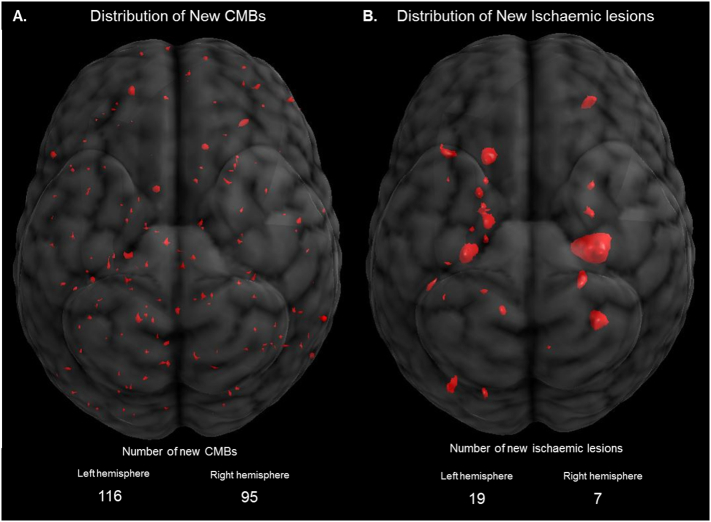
Positions of (A) new cerebral microbleeds, and (B) new MRI FLAIR lesions seen in the combined imaging data for all 46 patients. Only 4 new lesions were observed in the right hemisphere MCA territory compared to 14 in the left MCA territory; consistent with fewer emboli identified entering the right MCA in the 1 min following release of the AxC (right: 601 emboli, vs. left 1247 emboli). A similar observation was made for the CMBs, with 38 found in the right MCA territory and 58 in the left MCA territory. Estimated total numbers of bubbles and volume of air entering the MCA territories were similar for both hemispheres.
